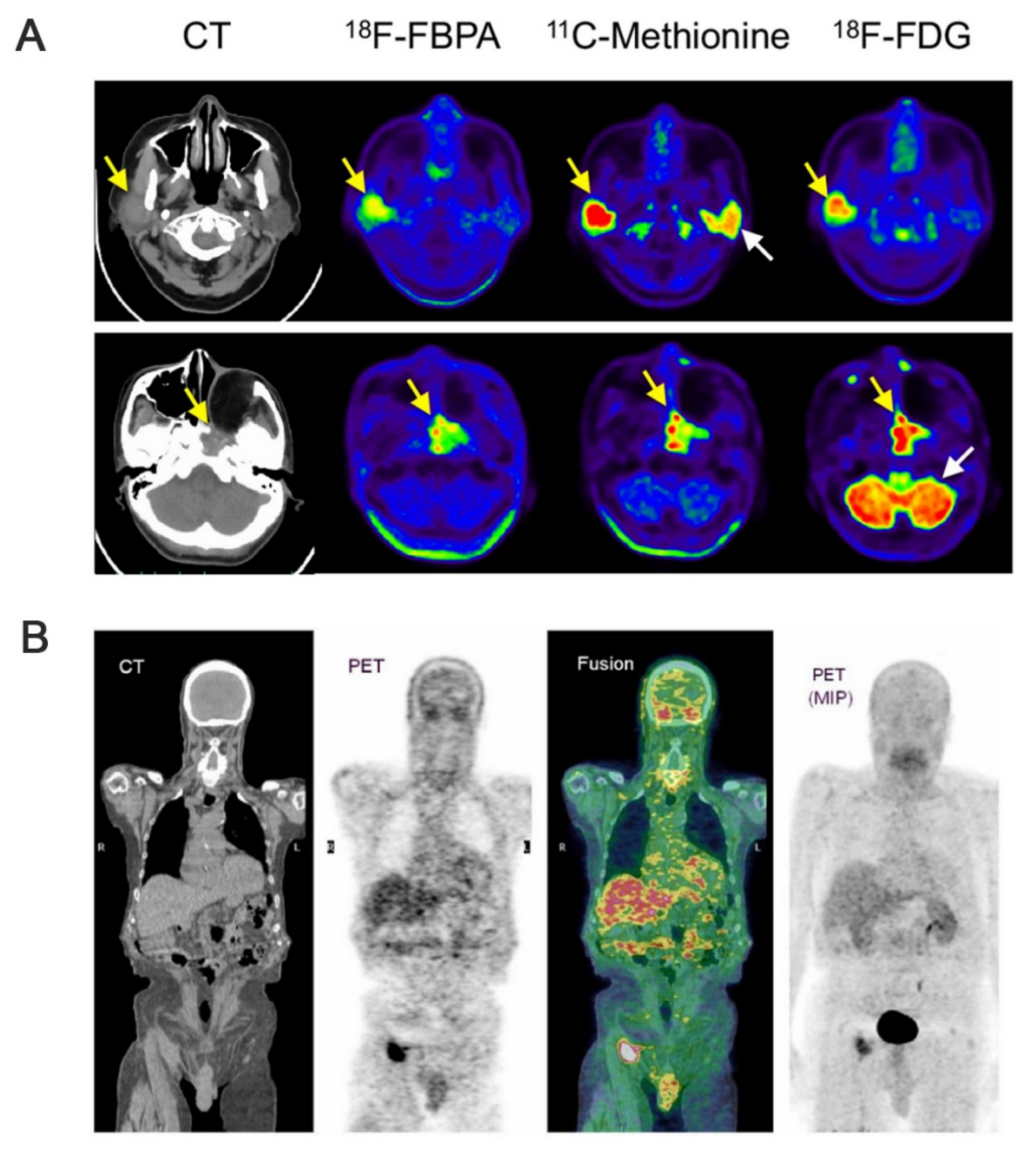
(A) PET/CT Images of Patients with Ductal Carcinoma (Upper Row) and Squamous Cell Carcinoma (Lower Row) of Salivary Glands Injected with F-FBPA and Other Radiation Agents. Reproduced with permission from Ref. 119. Copyright 2019, Springer Nature. (B) Coronal [^18^F] FBPA PET/CT images of a patient with melanoma in right ankle (not shown) metastatic to right inguinal lymph node (visible in all images). Reproduced with permission from Ref. 120. Copyright 2009, Elsevier.
